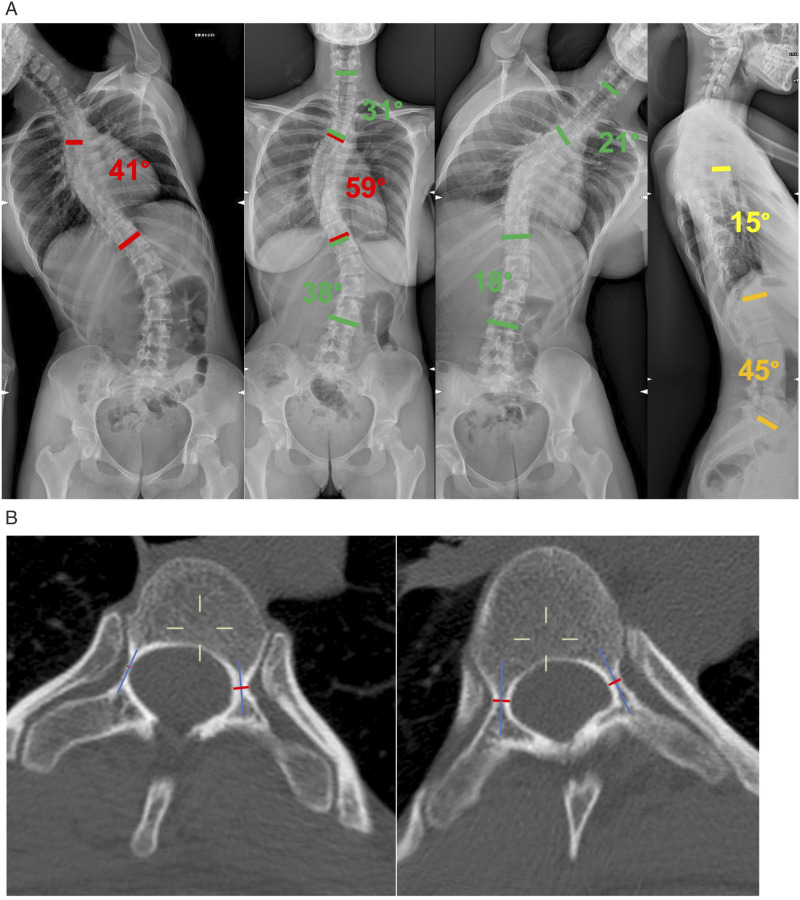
Exemplificative case. (A): Preoperative radiographic appearance of a 20 years old female patient with a 59° main thoracic curve. The side bending and lateral films, reveal the unstructural nature of the proximal thoracic and of the lumbar curves (B): Axial CT-scan of apical vertebra of the PT curve (T1, left) and of the MT curve (T7, right). In blue, the pedicle axis, in red the PW (pedicle width). It can be noted the severe pedicle dysplasia in the PT curve (PWc = 0,4 mm; PWv = 2,8 mm) and the moderate dysplasia in the MT curve (PWc = 2,4; PWv = 2,9 mm).
